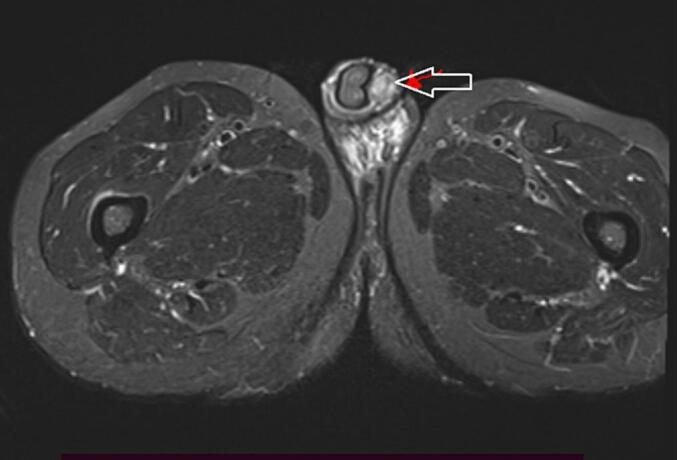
Axial pelvic MRI arrow head showing T2 hyperintense round lesion at distal penile invading corpus spongiosum.
